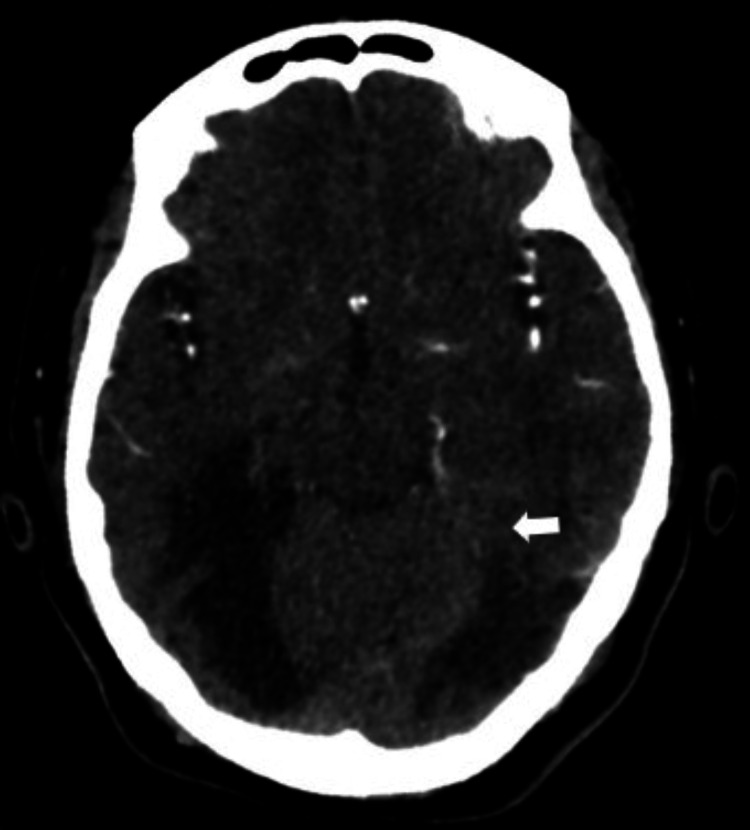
Cerebral CTA in the axial plane showed reduced opacification over the P4 segment of the left PCA (white arrow). CTA: computed tomography angiography; PCA: posterior cerebral artery.
